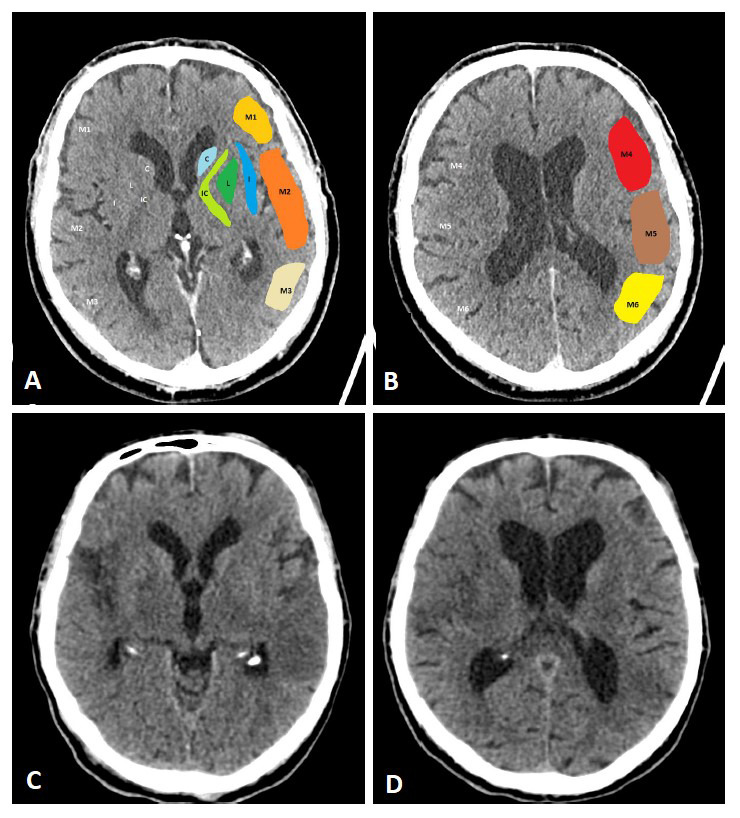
ASPECTS. Visualization of the ASPECTS territories (A,B). The
